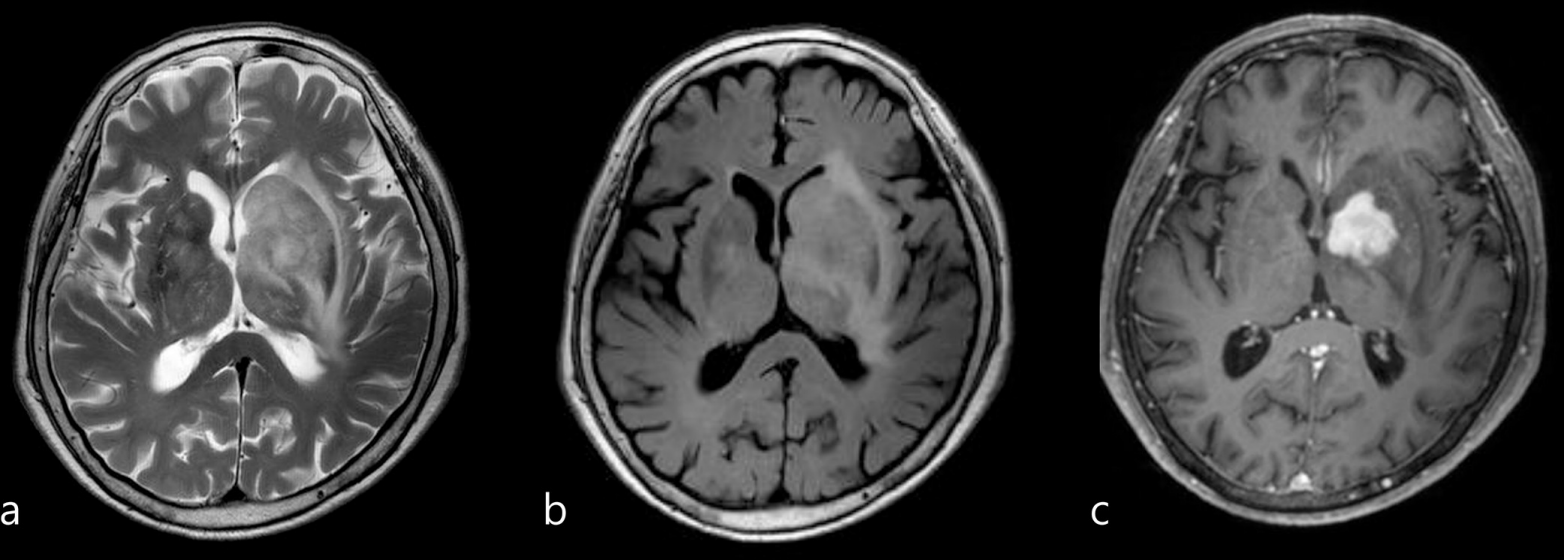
Images in an 86-year-old female patient presented with rapidly progressive dementia. Infiltrative high signal intensity lesion was noted on axial T2 weighted image (a) and FLAIR (b) with mass effect and edema involving left basal ganglia. Homogeneous enhancing mass was noted at the left basal ganglia on axial contrast-enhanced T1 weighted images (c). Lymphoma was suggested with the aid of contrast enhancement study rather than high grade glioma.
